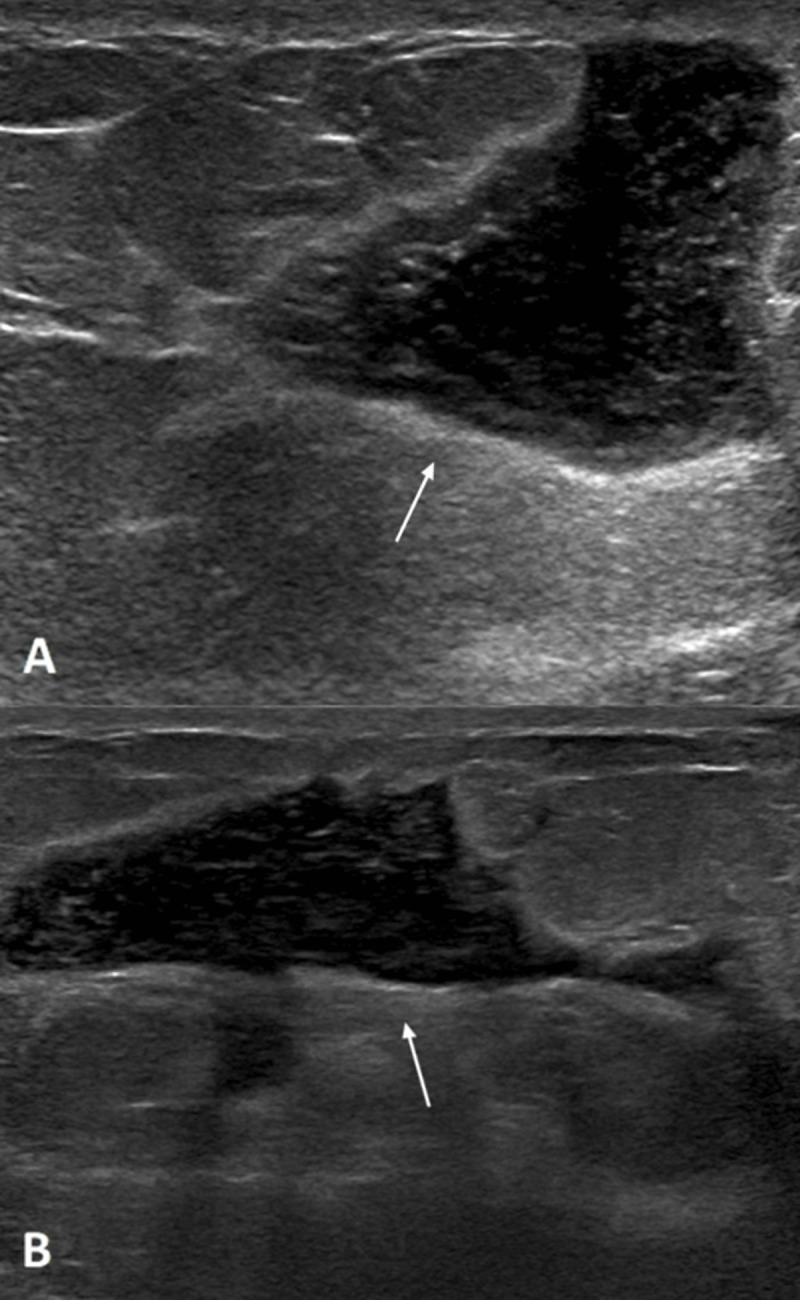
Magnetic resonance imaging (MRI) demonstrated round and oval masses with smooth margins and peripheral enhancement in both breasts on contrast-enhanced subtraction images (*Figure 3A* and *3B*, white arrows). Diffusion restriction was observed in all lesions (DWI; *Figure 3C* and *3D*, white arrows). The overlying skin and areola were thickened.
